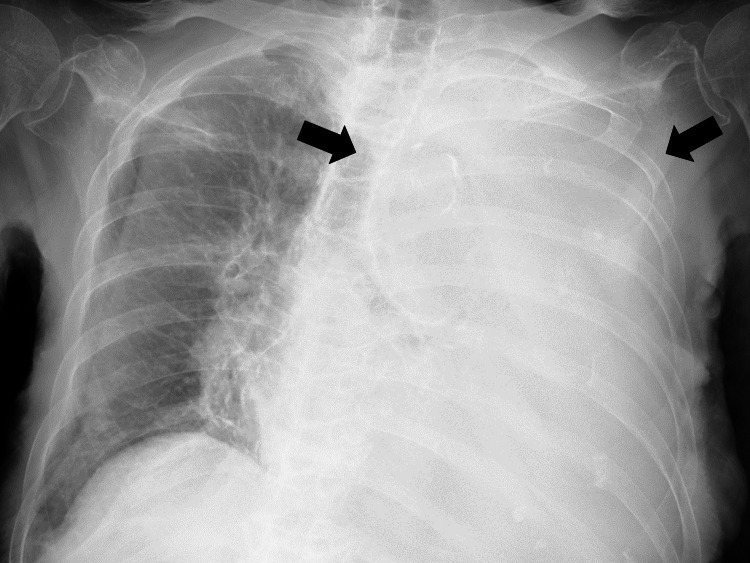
Chest X-ray showing left pleural effusion (black arrows)
